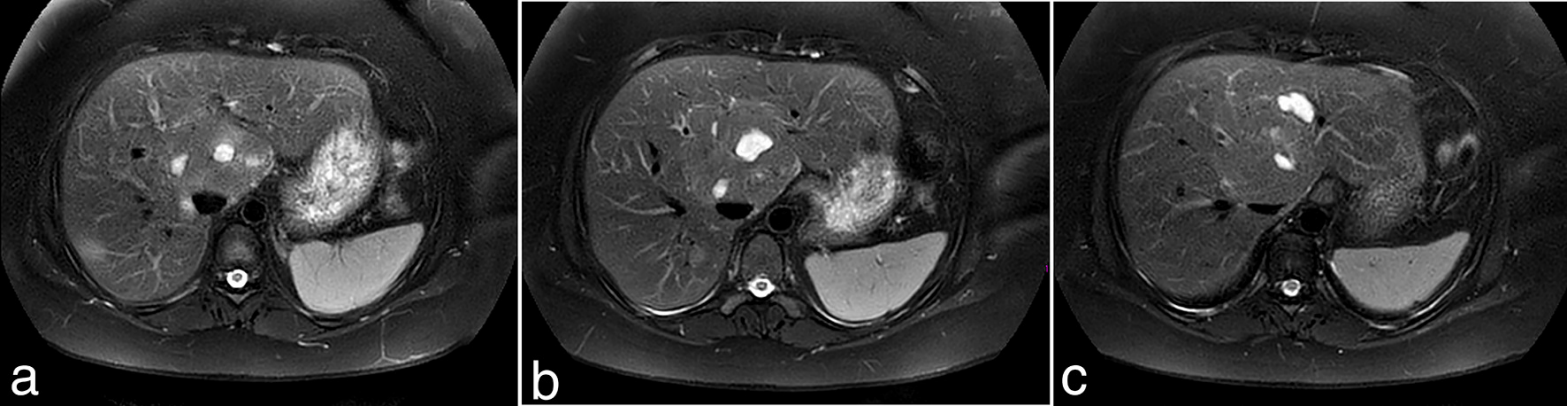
Consecutive slice through the liver on follow-up MRI (*T*_2_ SPAIR) in September 2016 demonstrates multifocal high signal within the dominant adenoma.
